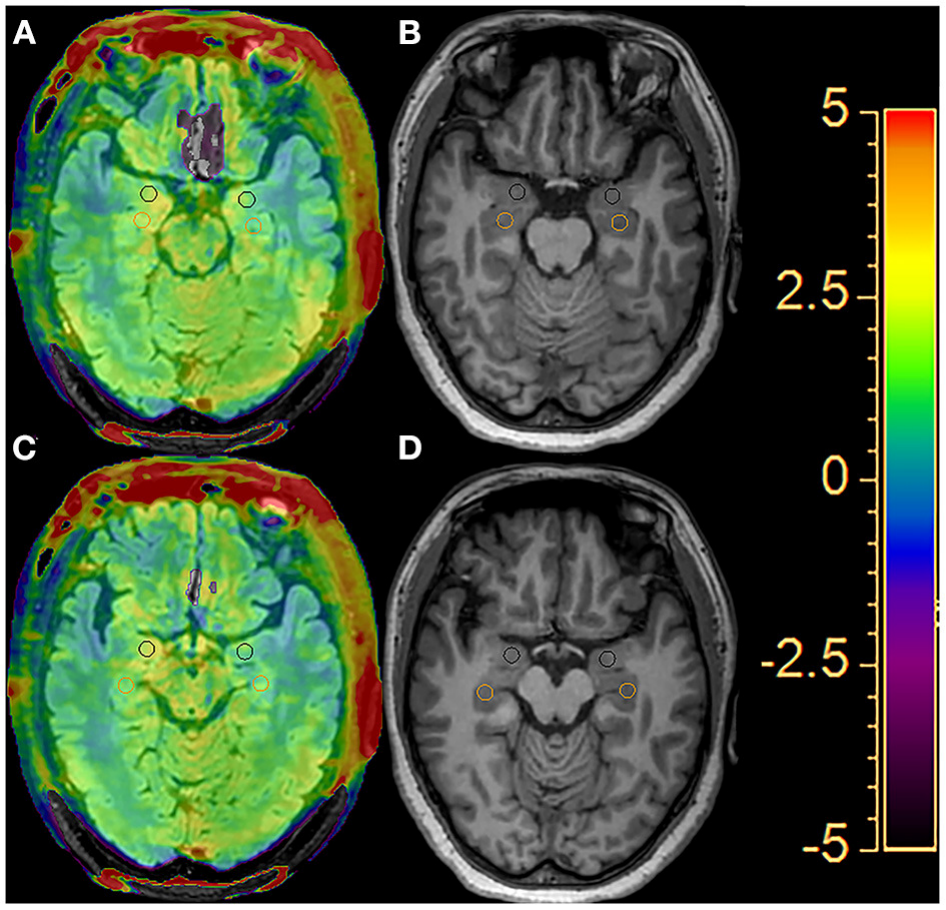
An example of the definition of the regions of interest (ROIs) of the bilateral hippocampus (orange circle) and amygdala (black circle) for quantitative analyses. Two consecutive layers of the FLAIR image with APTw overlay (A, C) and the T1-weighted image (B, D).
